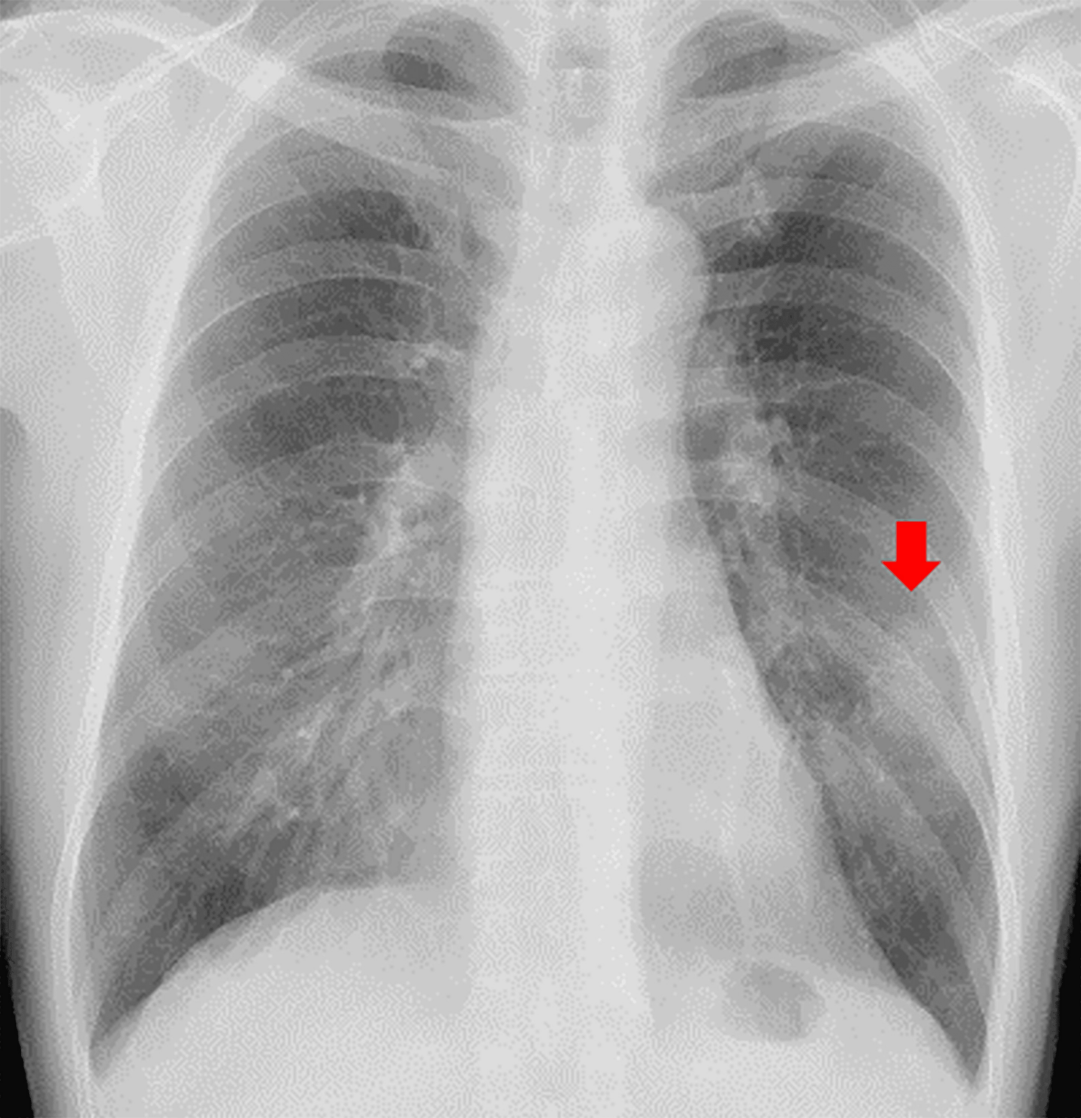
Chest X-ray findings The chest X-ray revealed a 3 cm mass in the left middle lung field.
